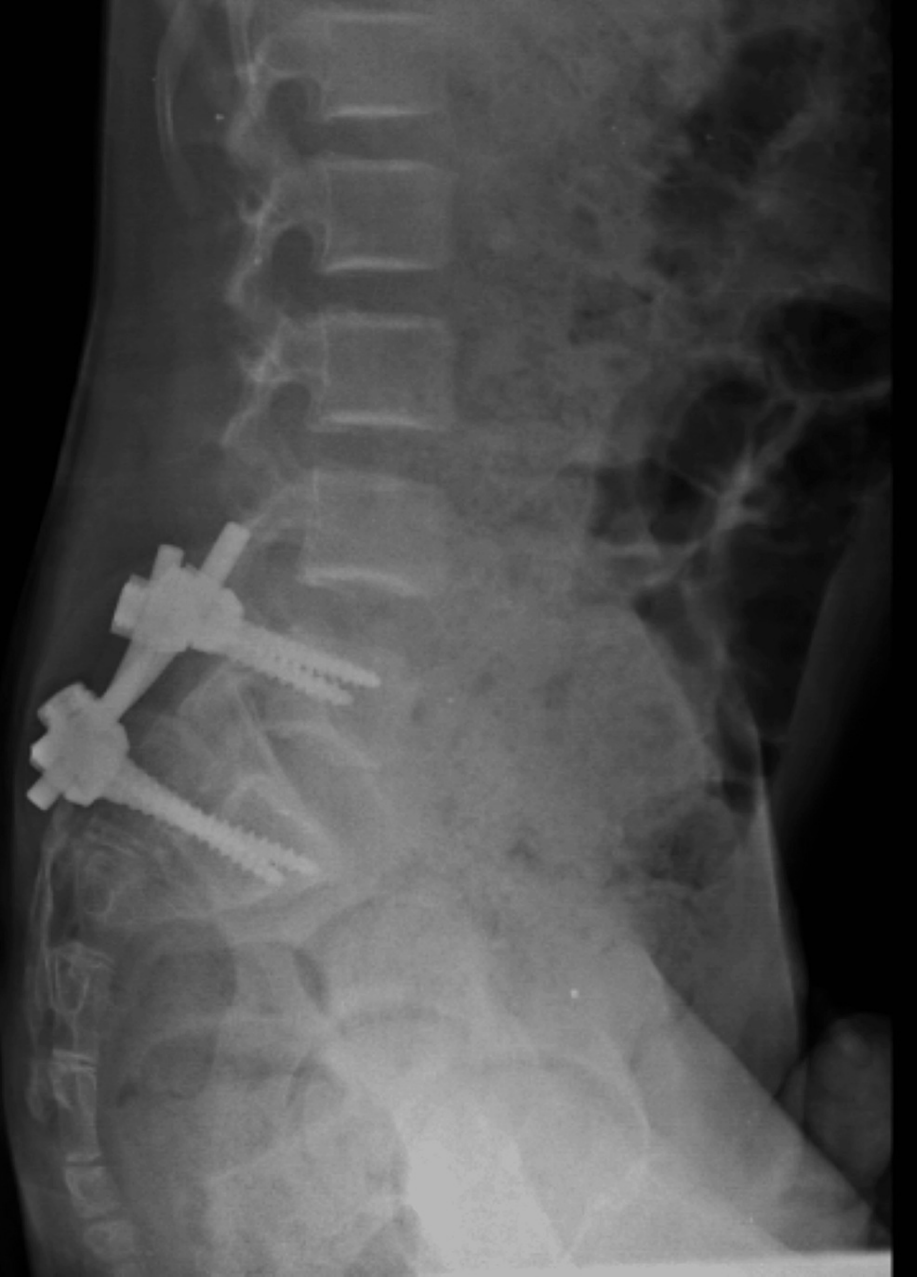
Post-operative lateral X-ray of the lumbar spine, showing spine fusion of the L4-S1 vertebra
